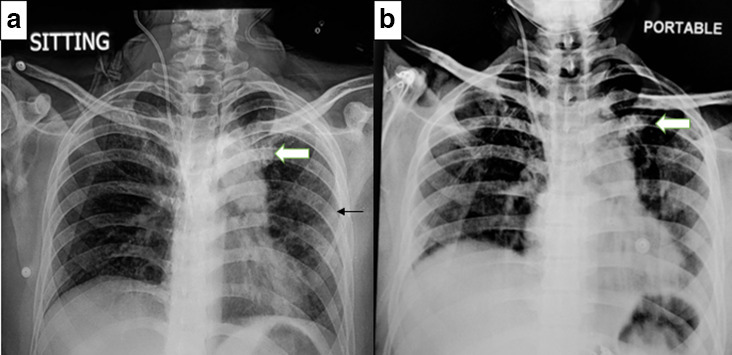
Frontal chest radiograph taken on Day 1 of admission (a) shows a patchy area of fibrosis in the left upper zone (white block arrow) with fine linear opacities in the left hemithorax with subpleural predominance (black arrow). Serial radiograph taken on Day 4 of admission (b) shows minimal increase in the size of the lesion (white block arrow).
